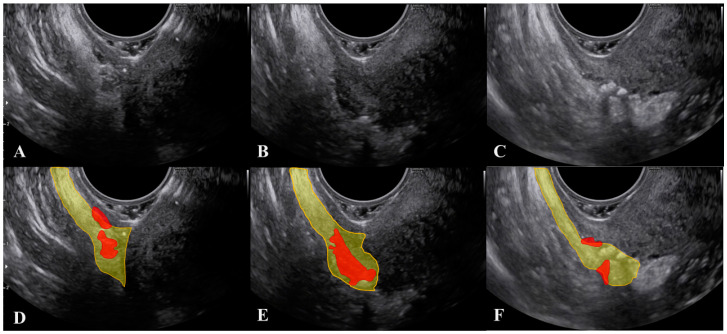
Modification of the visualization of lesions of the uterosacral ligament during the ultrasound when the probe is pushed. Figure 3 (D–F) represent the marked images respectively of figure (A–C). The uterosacral ligament is marked in yellow and lesions in red.
